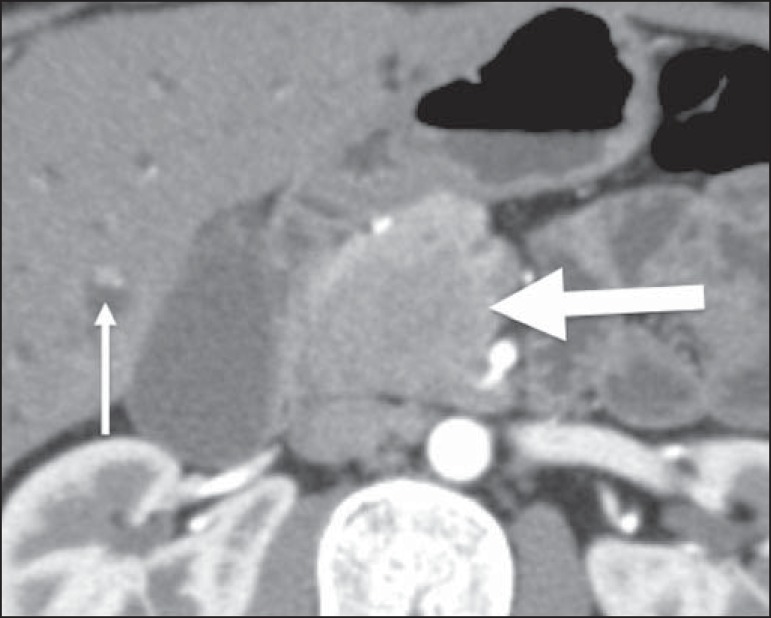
Pancreatic lymphoma. Contrast-enhanced CT of the abdomen, showing an
